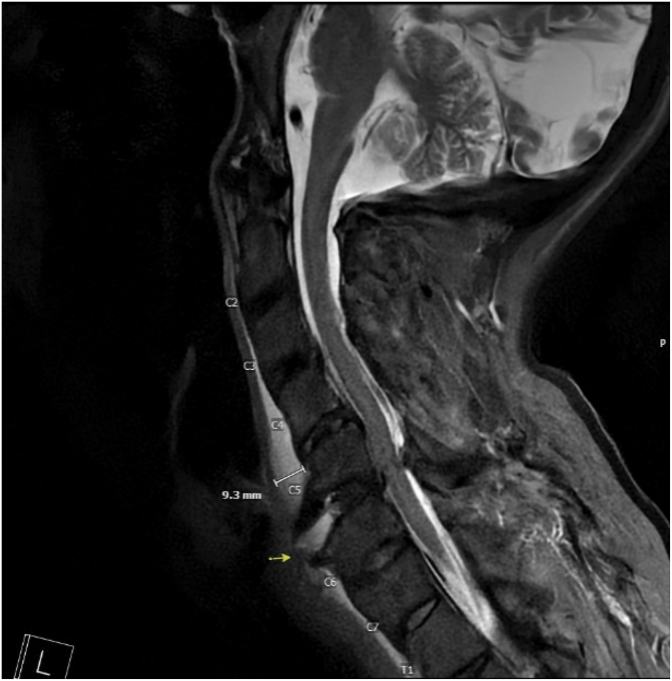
MRI Cervical spine w/o contrast demonstrating C5-6 traumatic grade 1 retrolisthesis with ligamentum flavum infolding/disruption.
